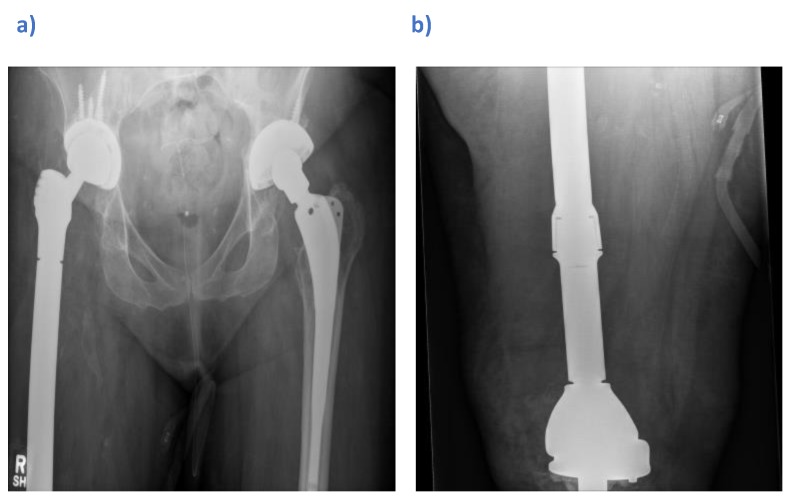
Post-operative radiographs showing a custom-made right total femoral replacement (Stanmore Implants Worldwide, Stryker) a) Anteroposterior view of the pelvis b) Lateral view of the femur
